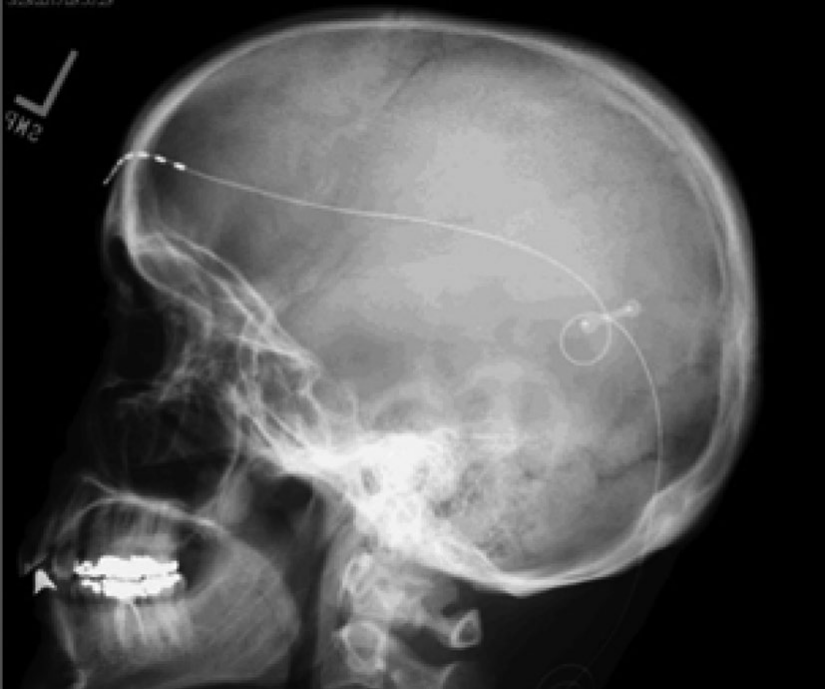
X-ray skull lateral view showing the supraorbital nerve stimulator in the supraorbital region with its anchor and strain-relief loop in the parieto-occipital region [26•]
